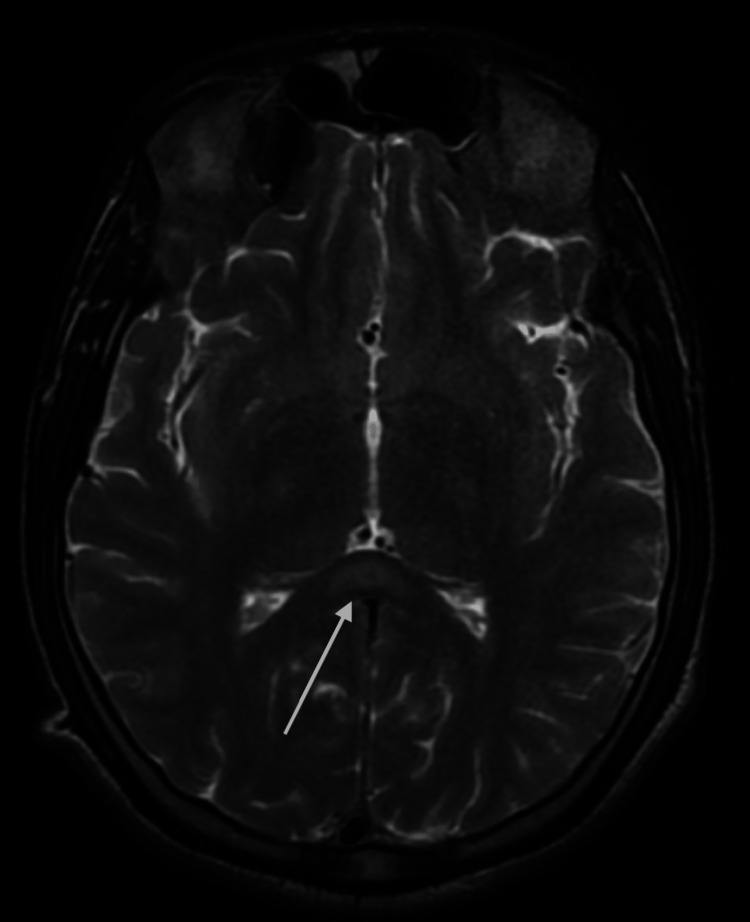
MRI imaging showing hyperintense signal on the T2WI T2WI: T2-weighted
